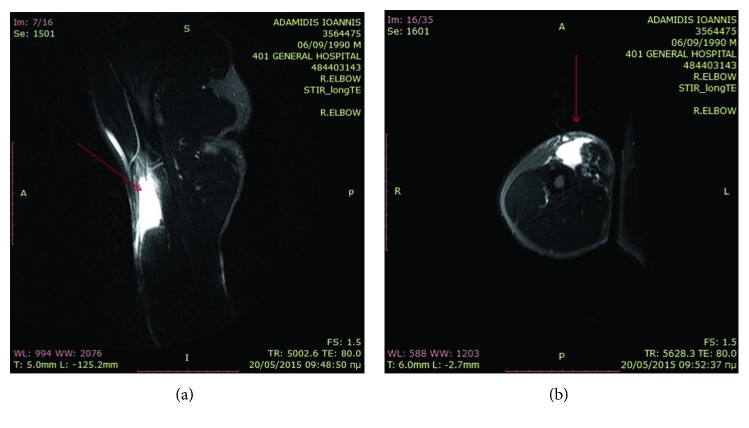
(a, b) MRI images showing an intrasubstance tear of the biceps brachii (arrows) with a large hematoma filling the defect.
